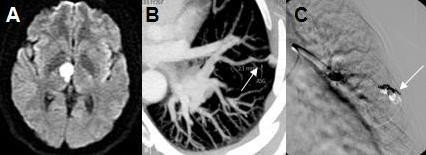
Radiological features of patient 2. A: Brain MRI diffusion hyperintensity in the right anterior-medial thalamus region; B: Contrast-enhanced thoracic CT scan shows PAVMs (arrow); C: Pulmonary angiography shows second PAVMs embolized (arrow).
